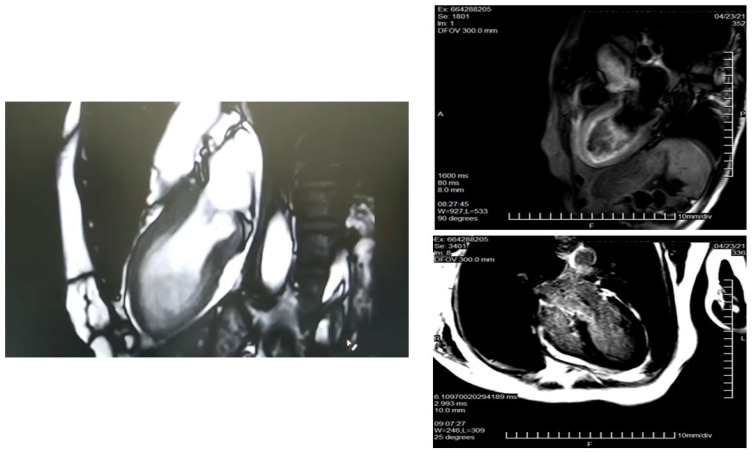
CMR in a patient with Takotsubo syndrome. Legend: Same patient as Figure 4. CMR study. Cine images with an apical ballooning. T2-weighted images showing myocardial oedema. There is no LGE. CMR: cardiac magnetic resonance; LGE: late gadolinium enhancement.
